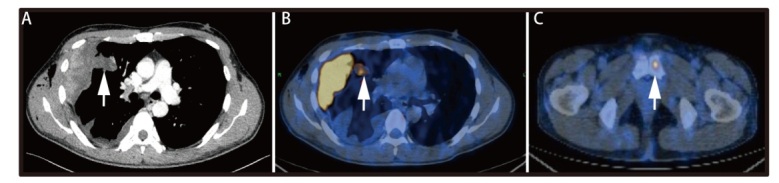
患者基线期影像学表现。A：增强CT示右肺病灶伴胸膜转移；B：PET/CT示右肺及胸膜转移病灶；C：PET/CT示左侧耻骨转移。
